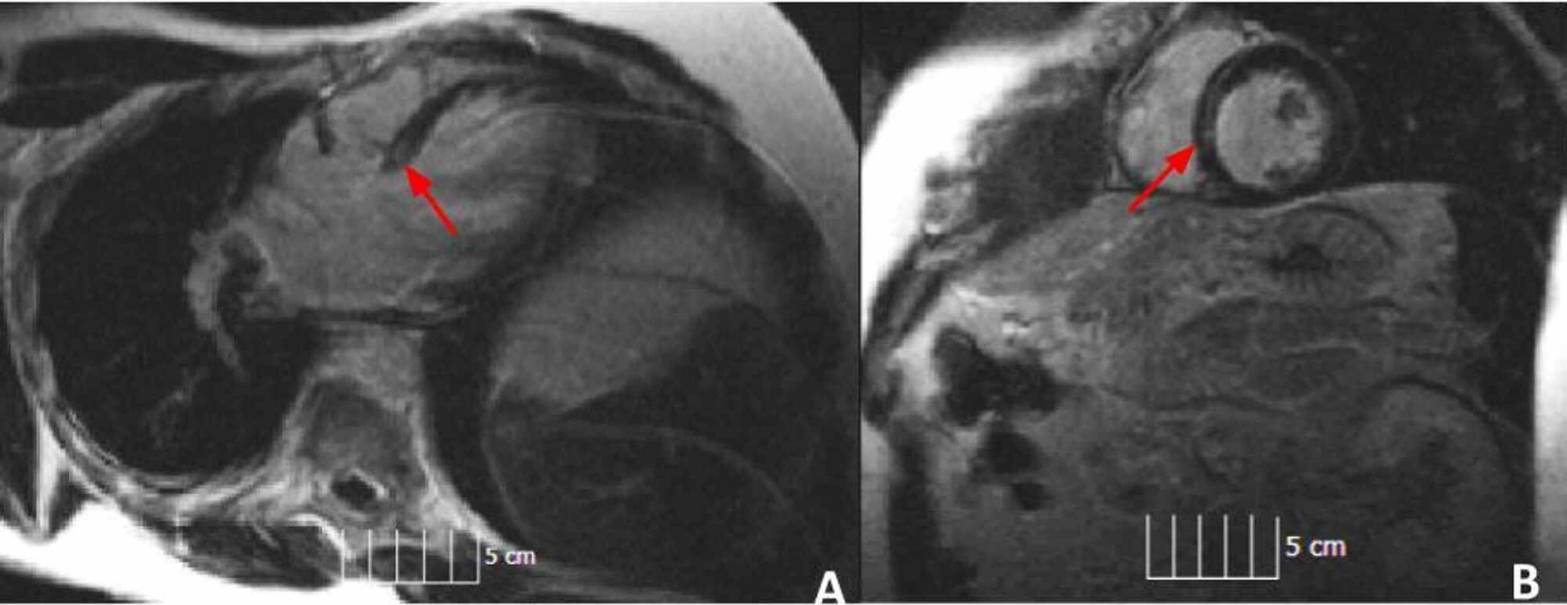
MRI of the heart showing fibrosis (red arrows) (A) Four chamber view. (B) Short axis view.
